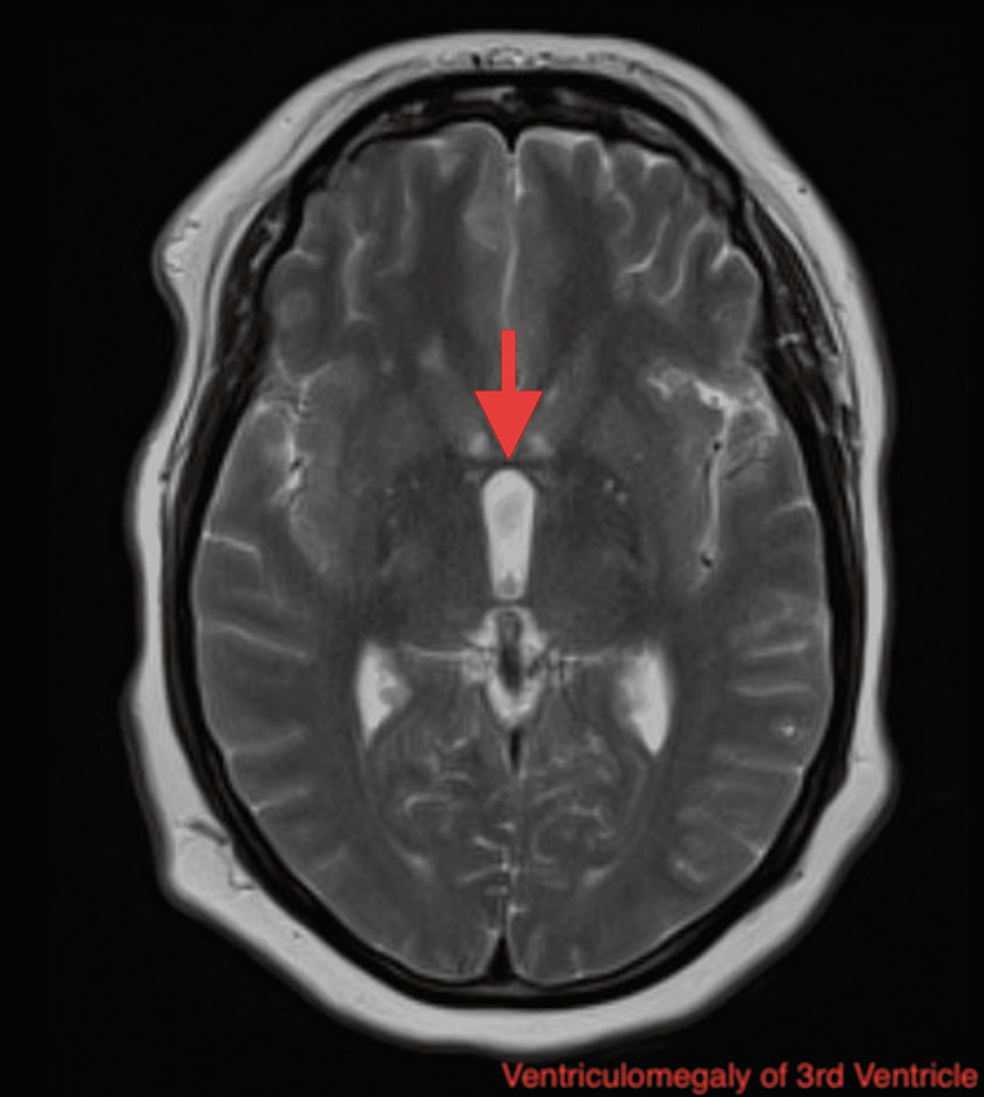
MRI of the head showing new ventriculomegaly of the third ventricle (arrow indicating hydrocephalus).
